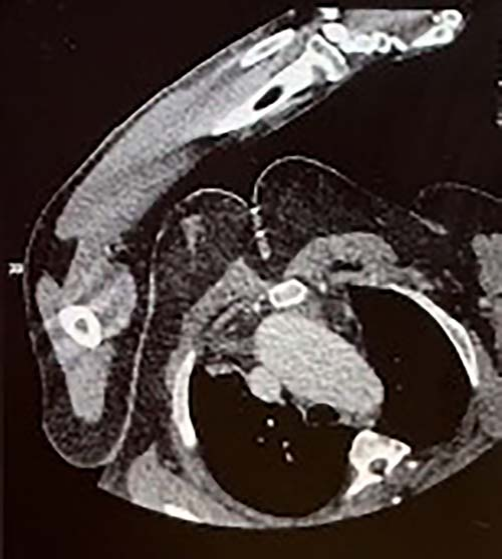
CT aorta thorax on patient’s re-admission four months later. Ascending aortic aneurysm has increased in size (measuring 60 × 61 mm) compressing the trachea.
